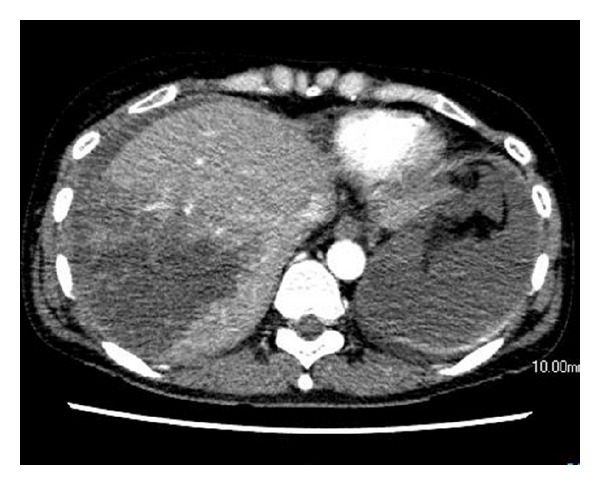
Enhanced CT on admission. Enhanced CT shows a deep liver laceration (Grade IV on the Organ Injury Scale of the American Association for Surgery of Trauma) without extravasation or pseudoaneurysm or arterioportal fistula formation.
